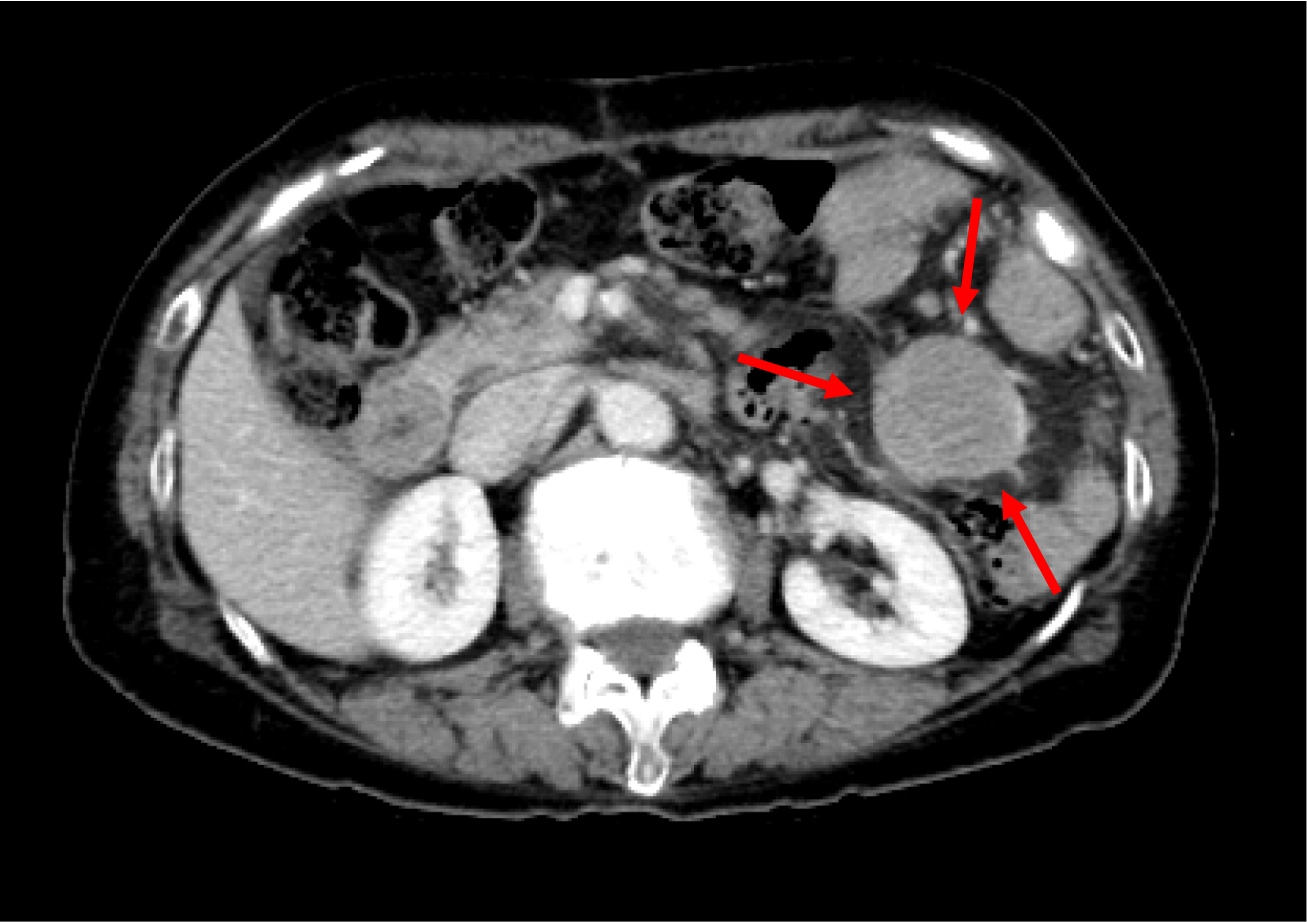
A CT scan shows progression of the residual lymphadenopathy three months after the operation
